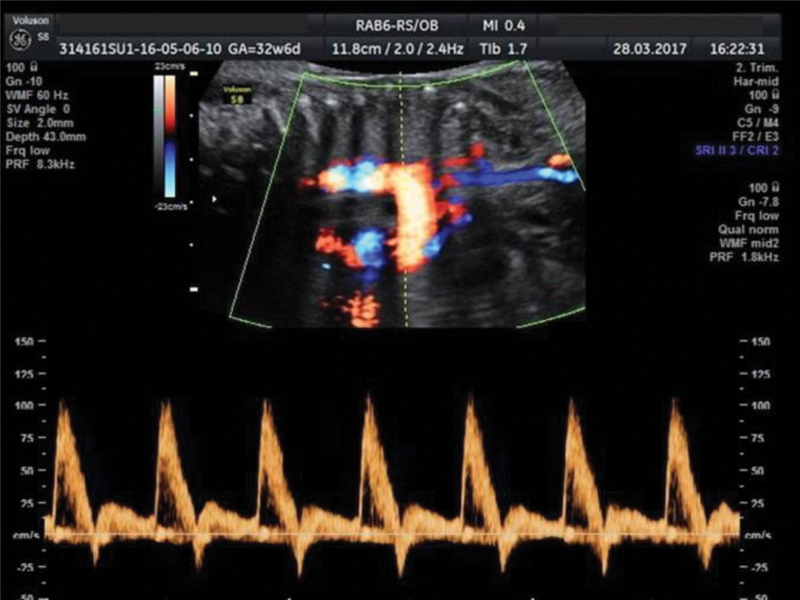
Aortic isthmus Doppler of a 32-week fetus revealing a brief reverse flow at the beginning of diastole.
